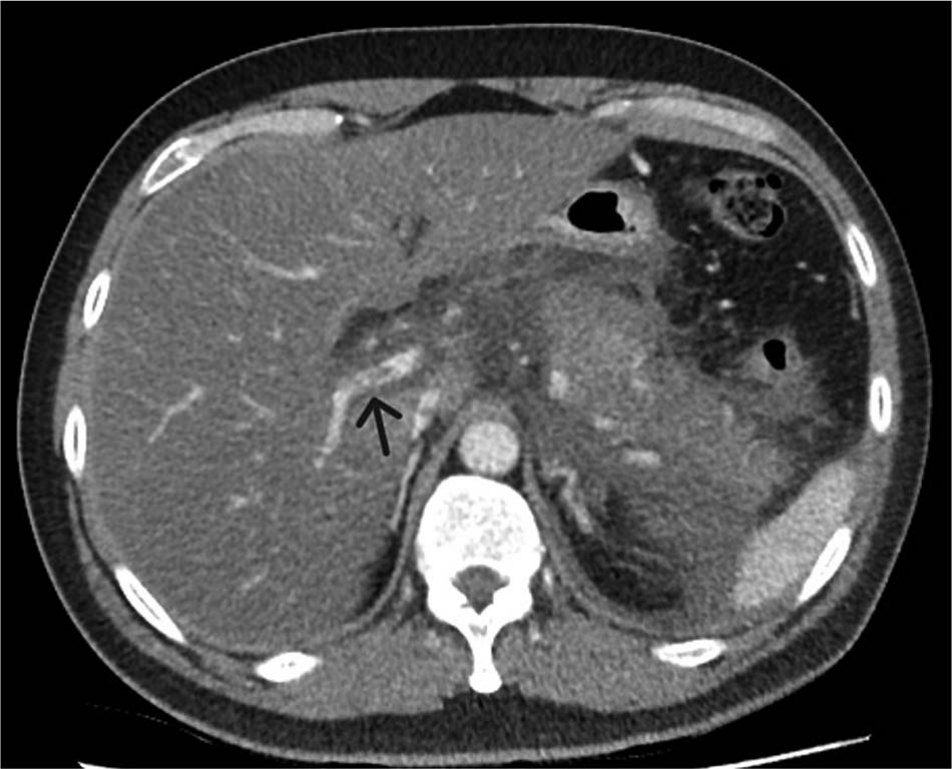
Axial CT image demonstrating non-occlusive portal vein thrombus (depicted by arrow).
